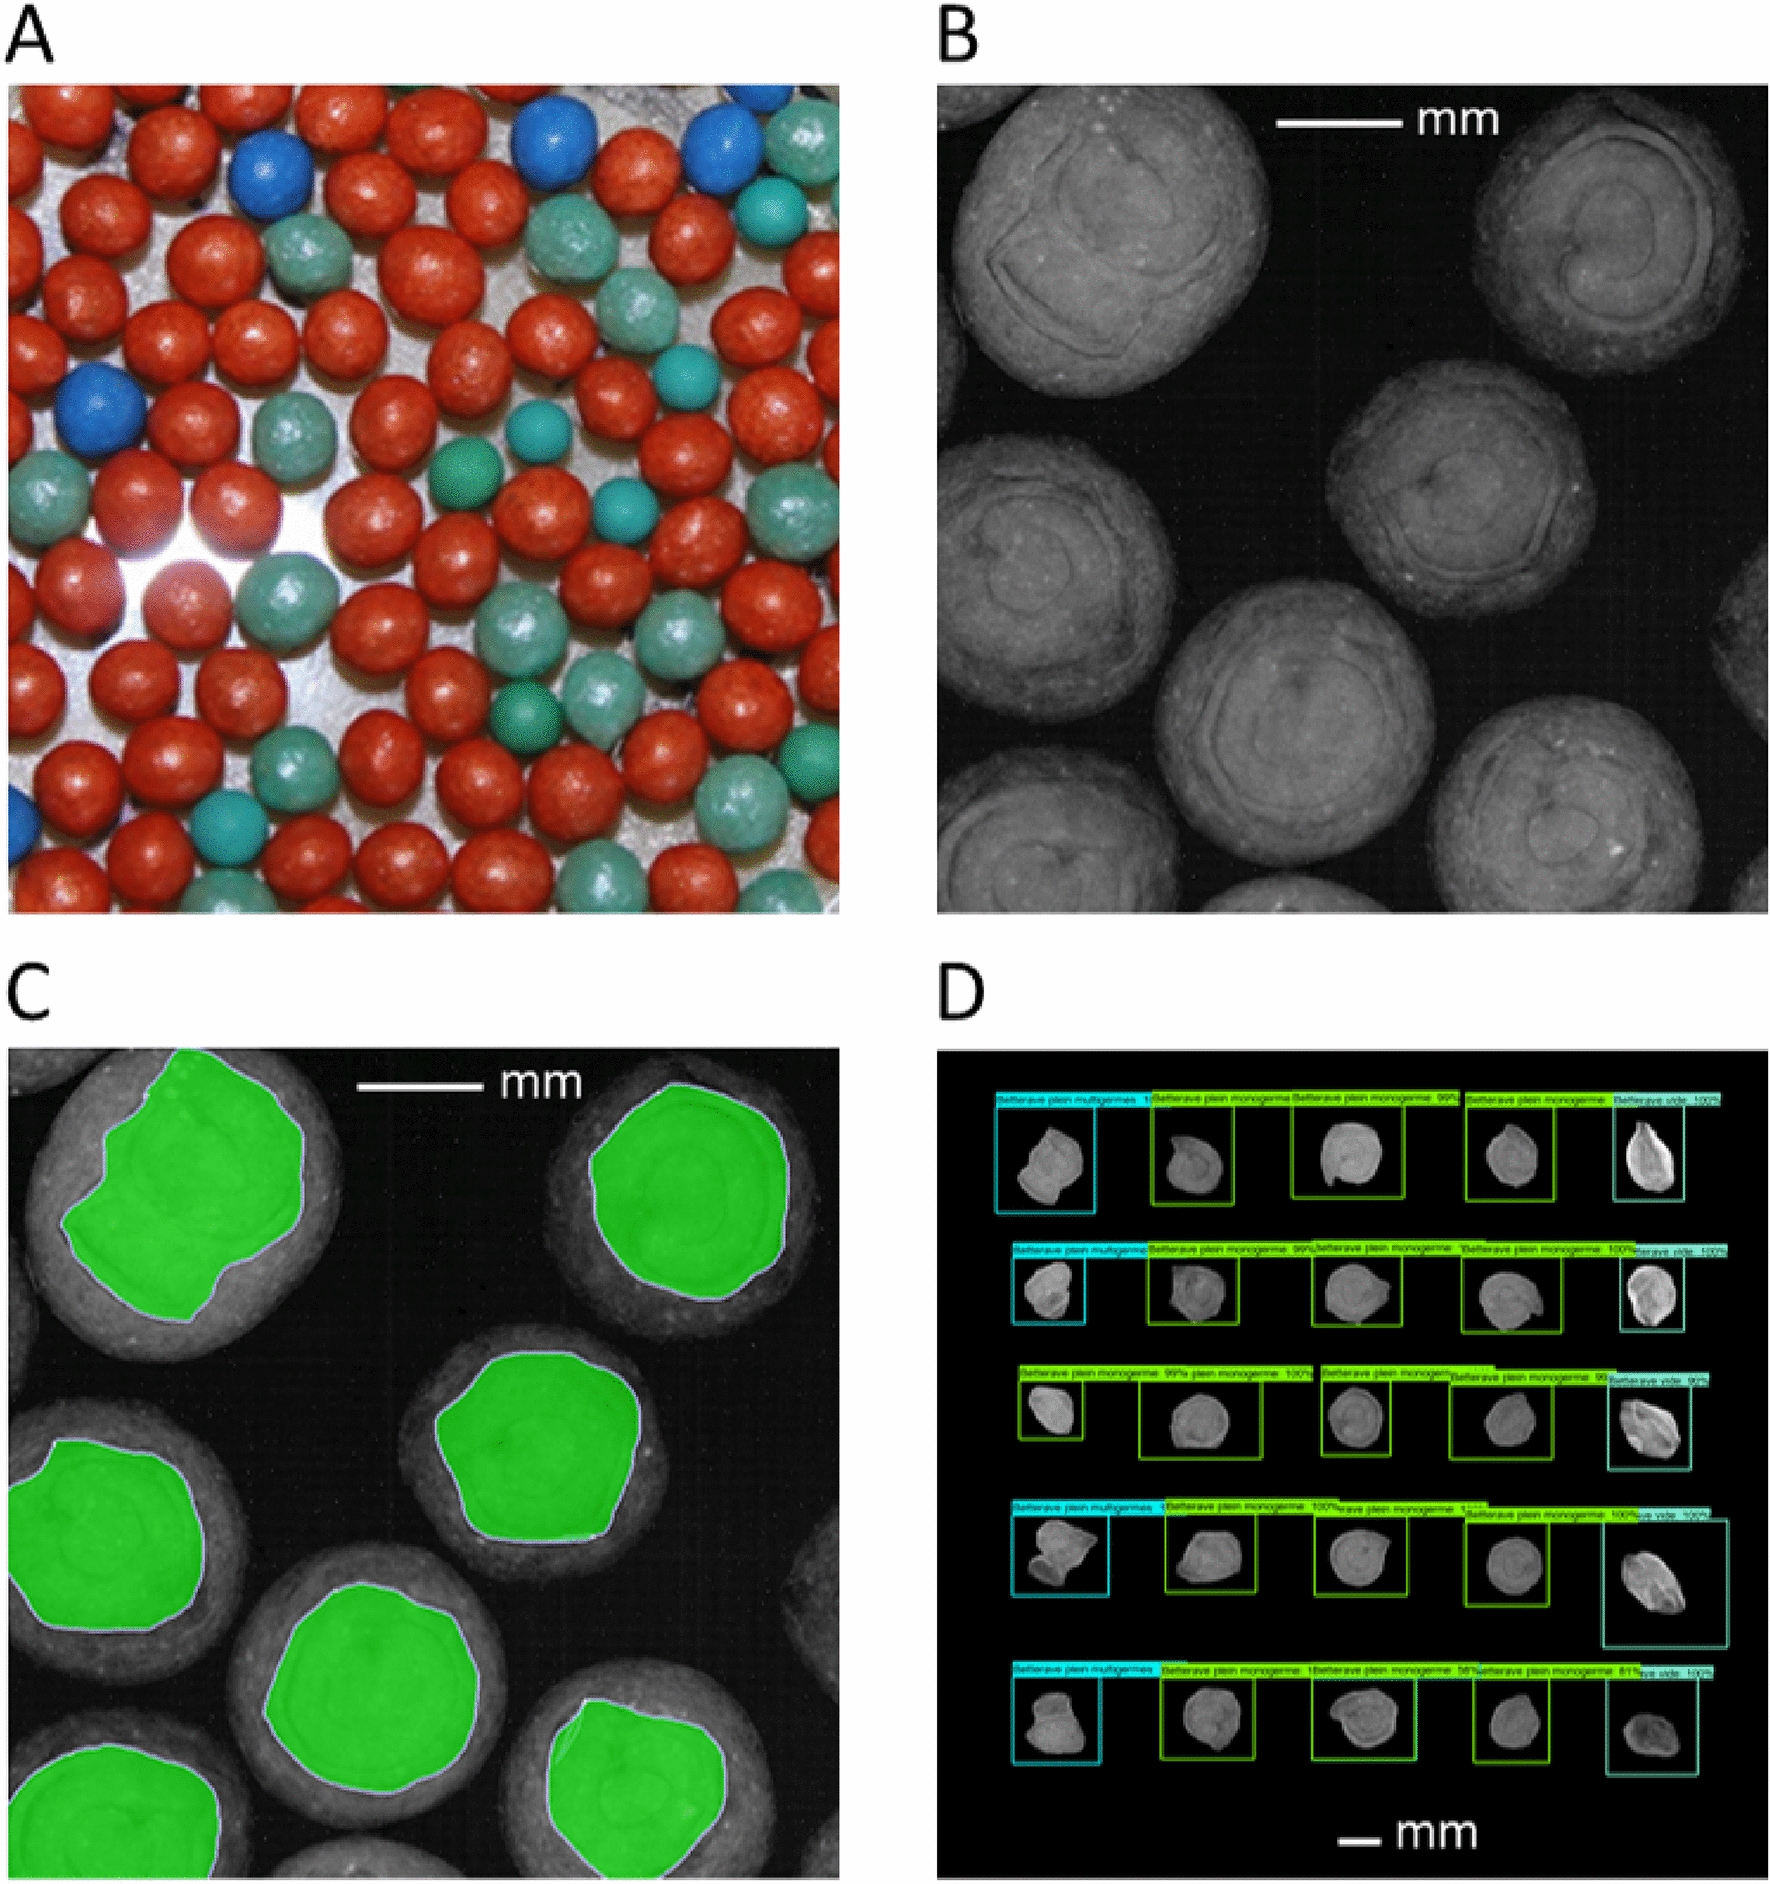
Detection robustness test on coated sugar beet seeds. (A) A sample of coated sugar beet seeds. (B) A snippet of an original 2D X-ray of coated sugar beet seeds. (C) Manual segmentation and extraction of the seeds. (D) Inference results of Faster R-CNN model reassembled 2D X-ray image
